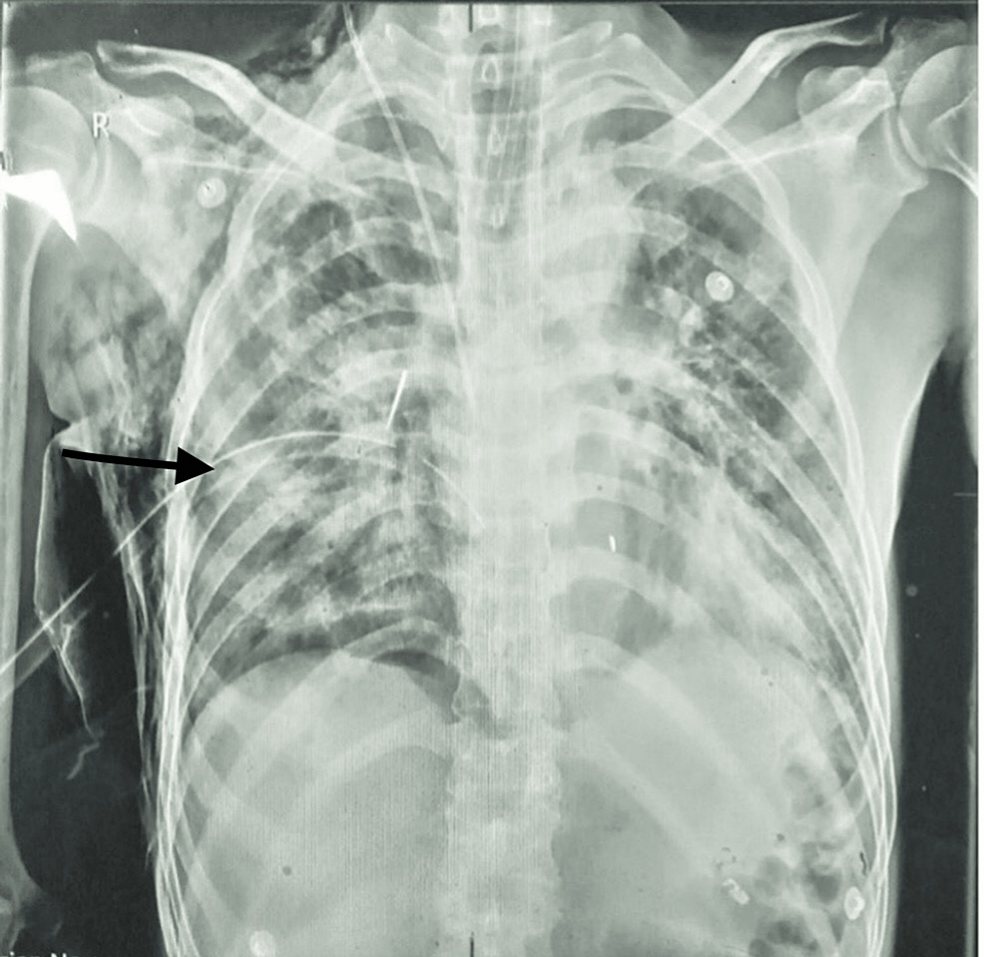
Subsequent chest X-ray after intubation and chest tube placement (black arrow).
